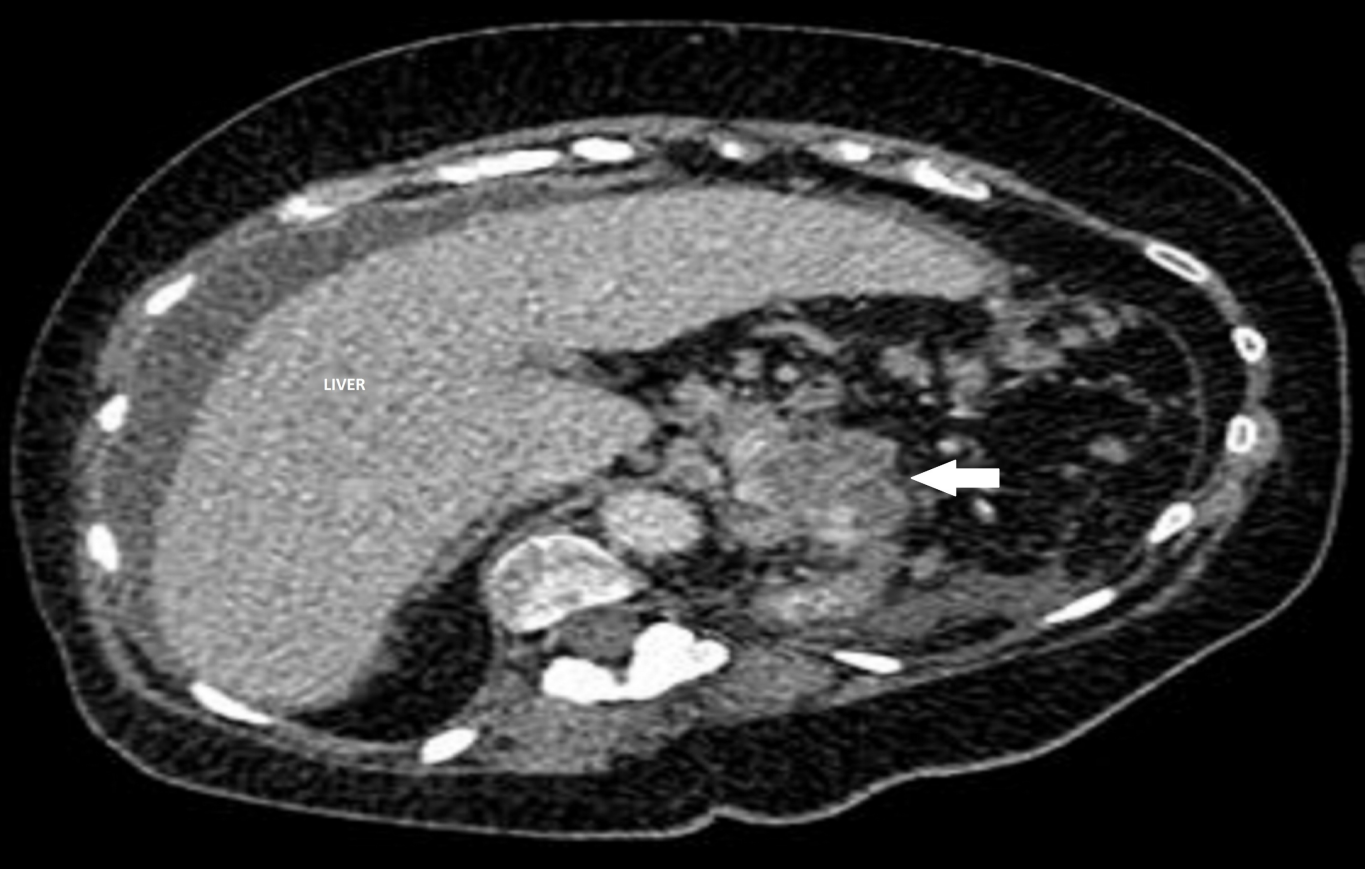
Contrast abdominal computed tomography (CT) scan. Axial view showing an irregularly marginated 3.0 x 3.0 cm heterogeneous hypodense mass (arrow) nearly completely replacing the distal pancreas, suspicious for pancreatic adenocarcinoma.
